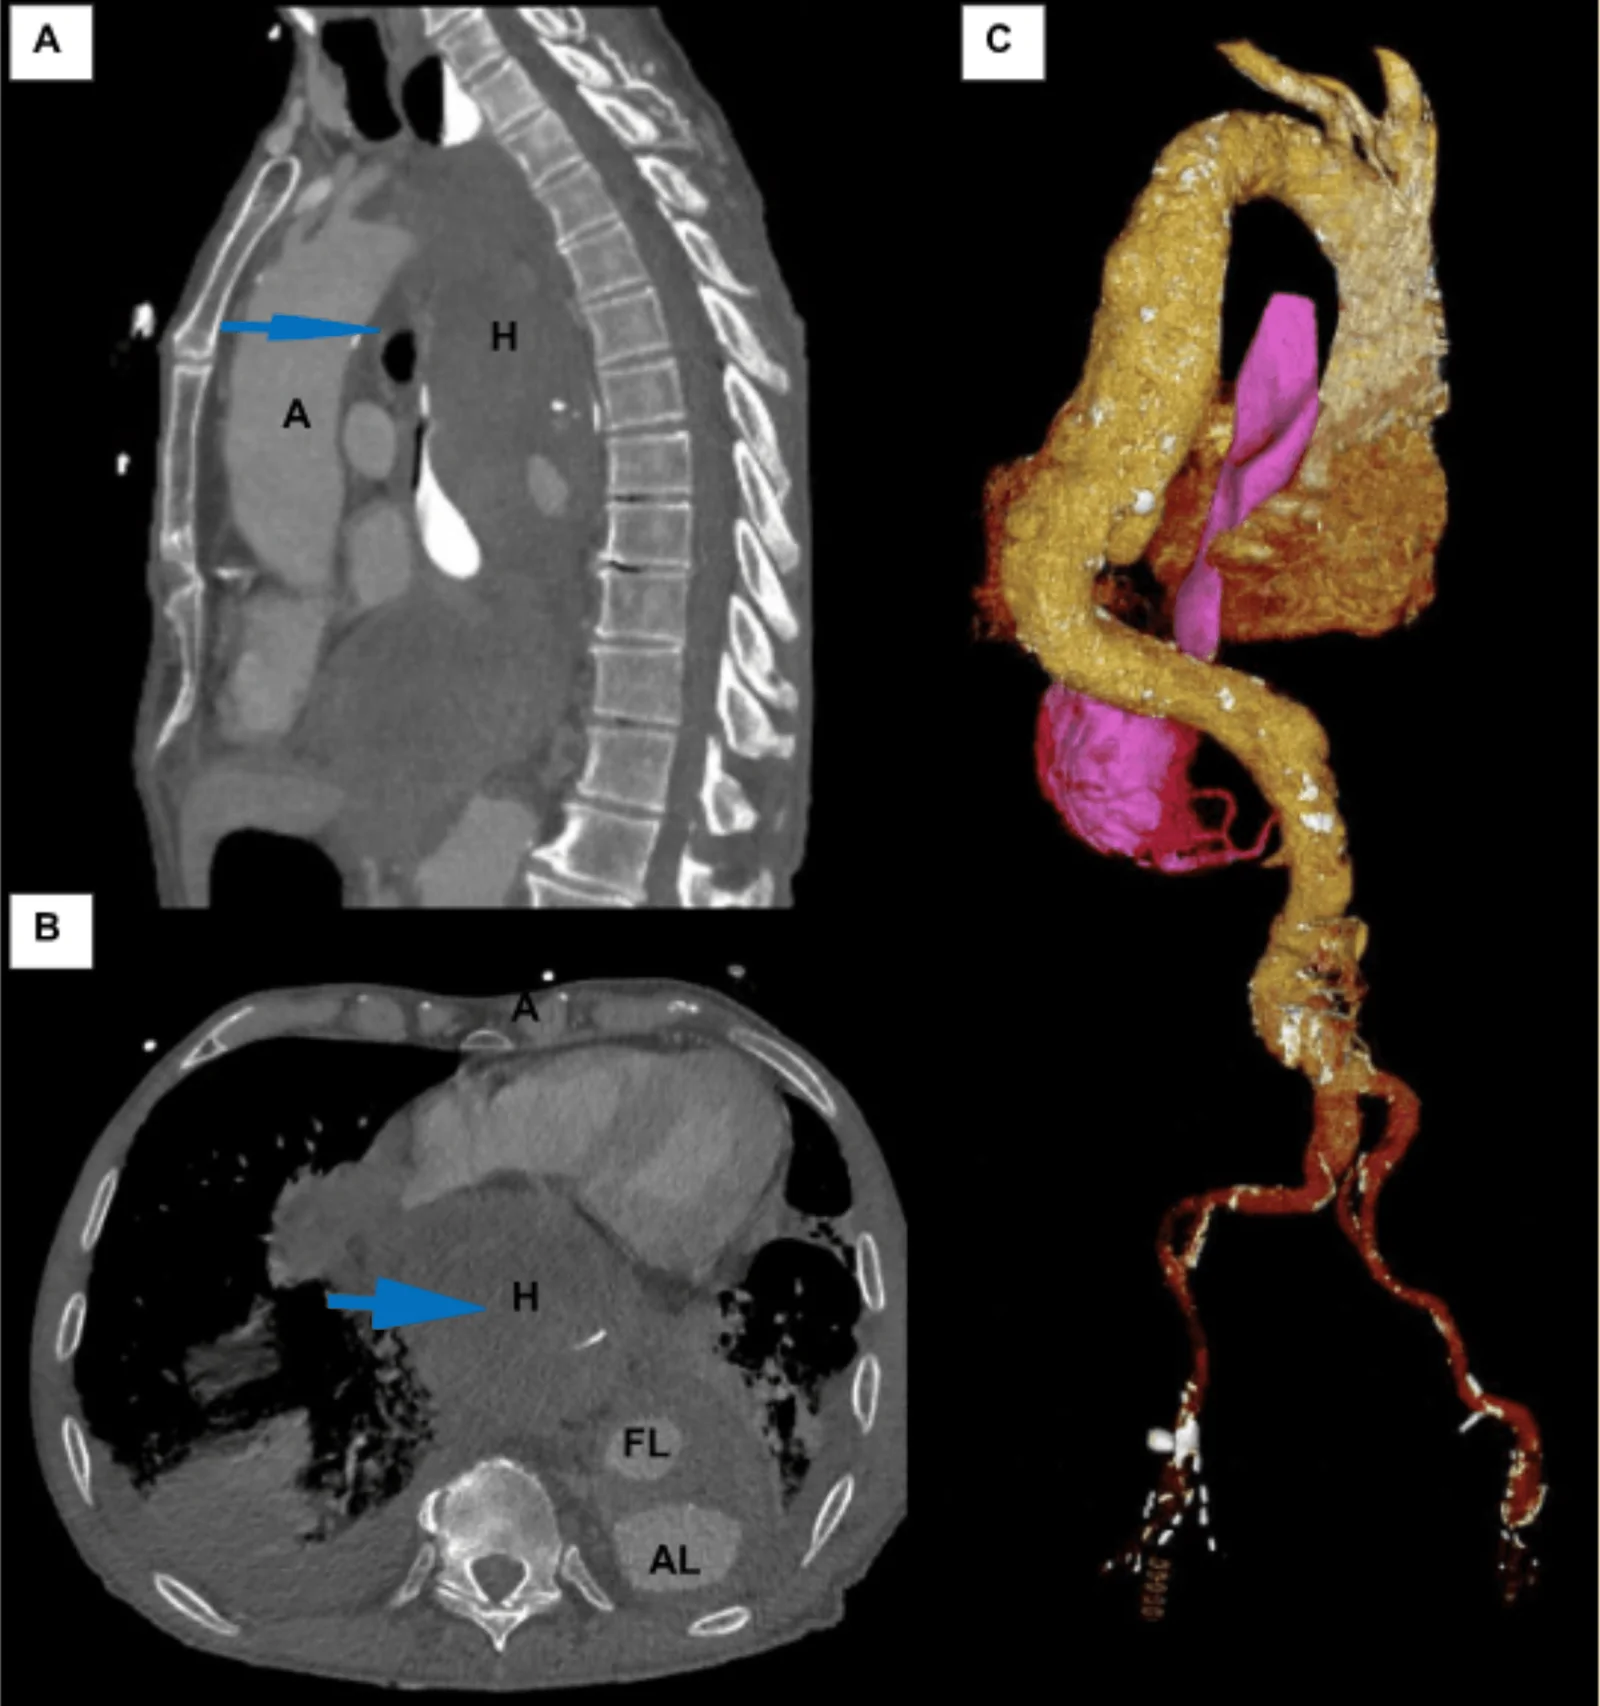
CT scan with gastrografin demonstrating thoracic hematoma and esophageal compression H: hematoma; A: aorta; H: hematoma; A: aorta; AL: aortic lumen; FL: false lumen (A) Sagittal reconstruction showing compression of the esophageal lumen by the mediastinal hematoma. (B) Axial reconstruction showing compression of the esophageal lumen. (C) Three-dimensional CT reconstruction of the aorta in a right posterior oblique view, with gastric contents opacified by contrast in pink
